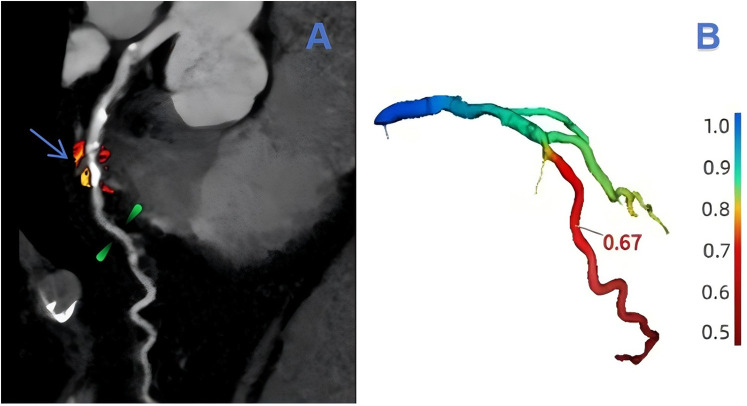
An example of target plaque selection in the left anterior descending branch (LAD, left anterior decending branch). In (A), the blue-arrowed portion is the area of severe stenosis in the LAD, the green-marked portion is 2 cm from the area of severe stenosis, and the red and orange areas are the outlined extent of lesion-specific peri-coronary adipose tissue. The value of CT-FFR at 2 cm from the region of severe stenosis can be obtained in (B) as 0.67.
